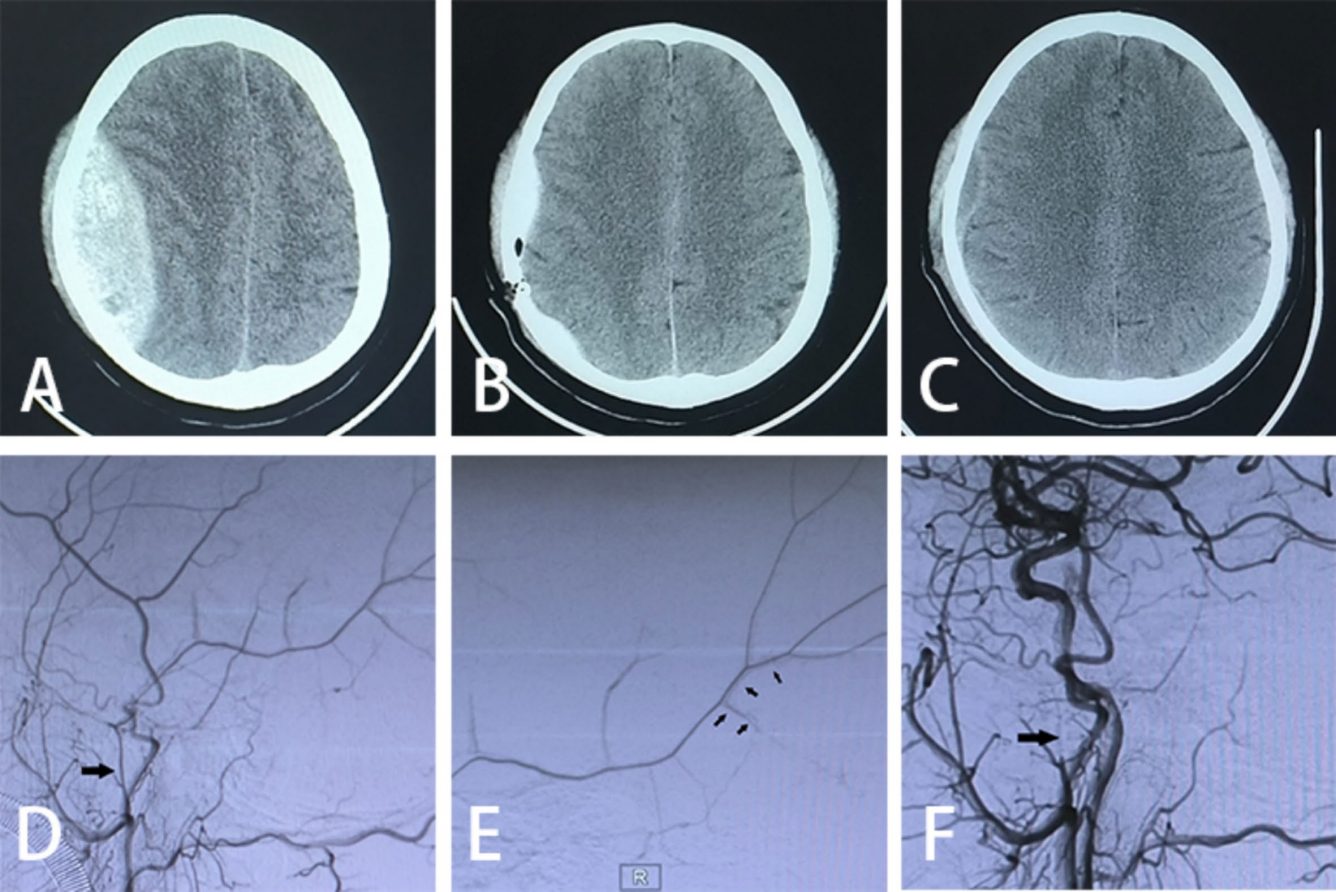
Imaging data. (A) Preoperative cranial CT scan; (B) postoperative cranial CT scan on day 1; (C) postoperative cranial CT scan on day 21; (D) preoperative DSA exhibiting MMA abnormality (large arrows); (E) intraoperative DSA demonstrating contrast spillage (small arrows); (F) postoperative DSA indicating undeveloped MMA (large arrows pointing to the spring-coil embolization).
